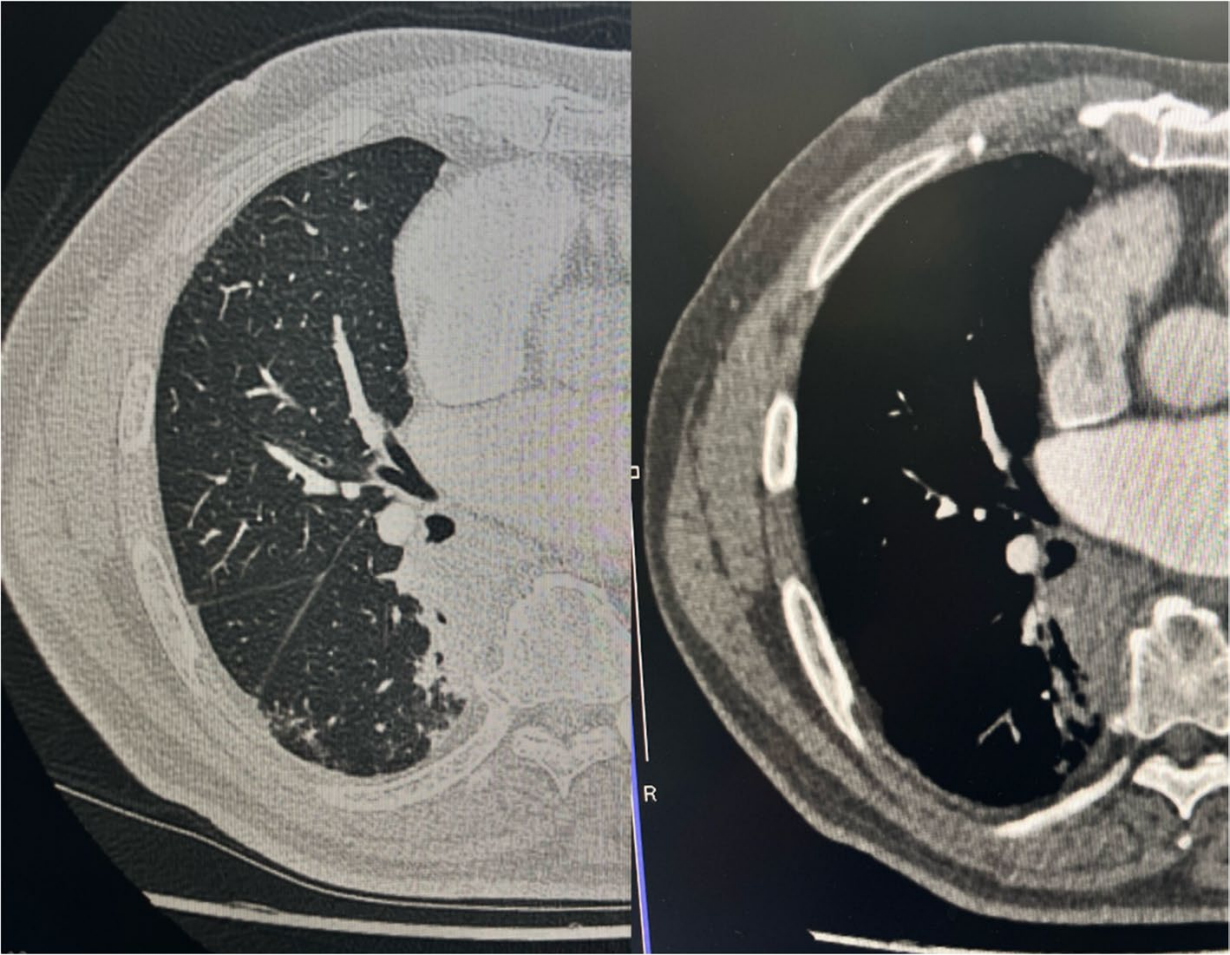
The computed tomography scan showed a tumor in the right lower lobe, measuring 43.0 × 40.0 × 36.0 mm. The cephalic side of the tumor extended to the middle lobar bronchus
